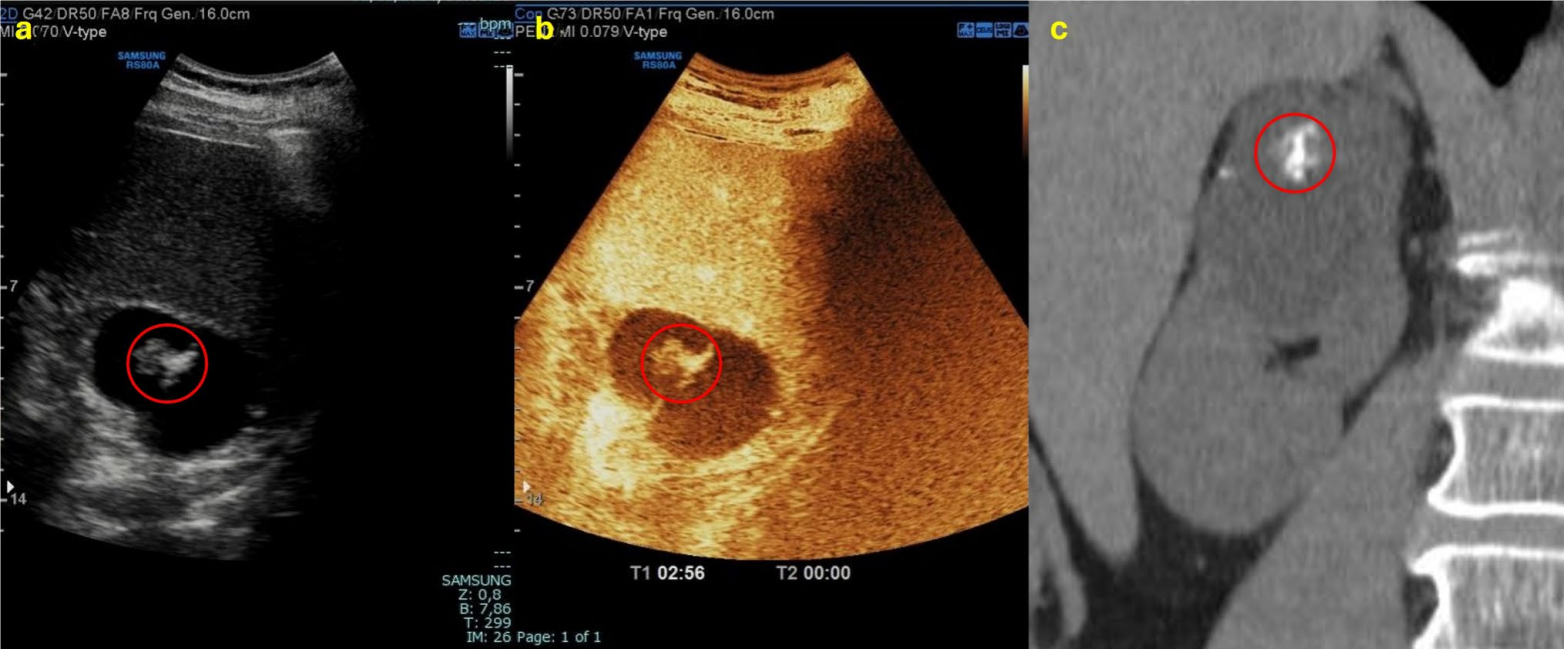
(a, b, c) B-mode US image a showed a cystic mass in the right upper pole of the kidney with hyperechoic content due to contextual calcifications (red circle); because of the calcifications, CEUS image b did not allow adequate evaluation of eventual enhancing septa; coronal non-contrast CT scan c confirmed the presence of calcifications but was not able to better characterize the mass. The mass was classified as Bosniak IIF and required further evaluation with MRI
